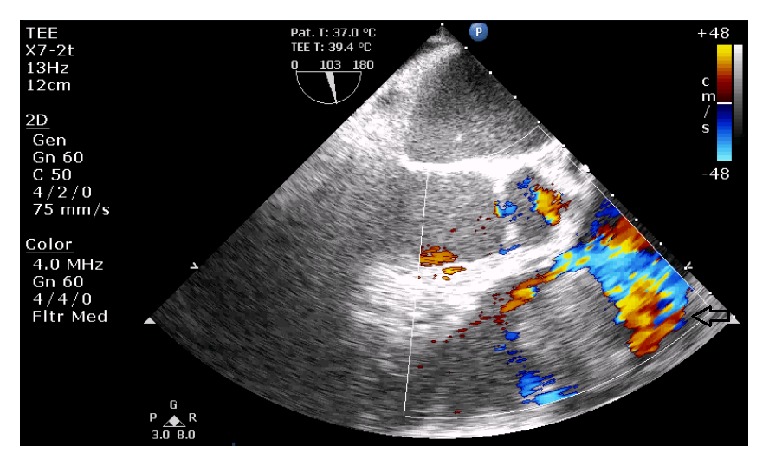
With the TEE on Doppler mode, we can estimate the tumour's blood flow obstruction in the pulmonary artery.
